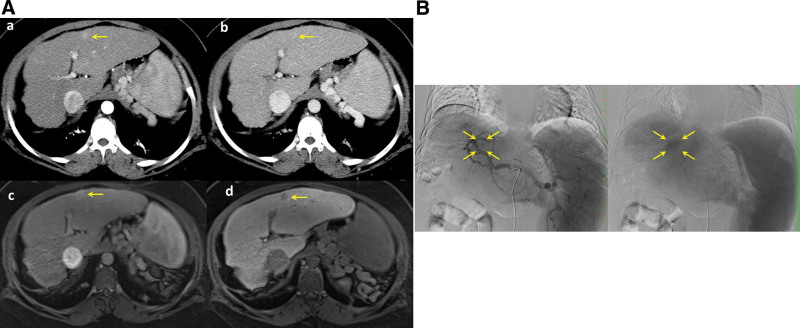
(A) Contrast enhanced abdominal CT and MRI at 19 y.o. (a, arterial and b, portal phase of CT; c, arterial and d, hepatobiliary phase of gadoxetic acid-enhanced MRI). The hypervascular nodule of 15 mm in diameter was located at the liver surface of the left lobe (arrows). (B) Hepatic arteriography from the celiac trunk. The nodule showed hypervascularity (arrows). From these findings, the tumor was diagnosed as hepatocellular carcinoma, and transarterial chemoembolization was performed. CT = computed tomography, MRI =  magnetic resonance imaging.
